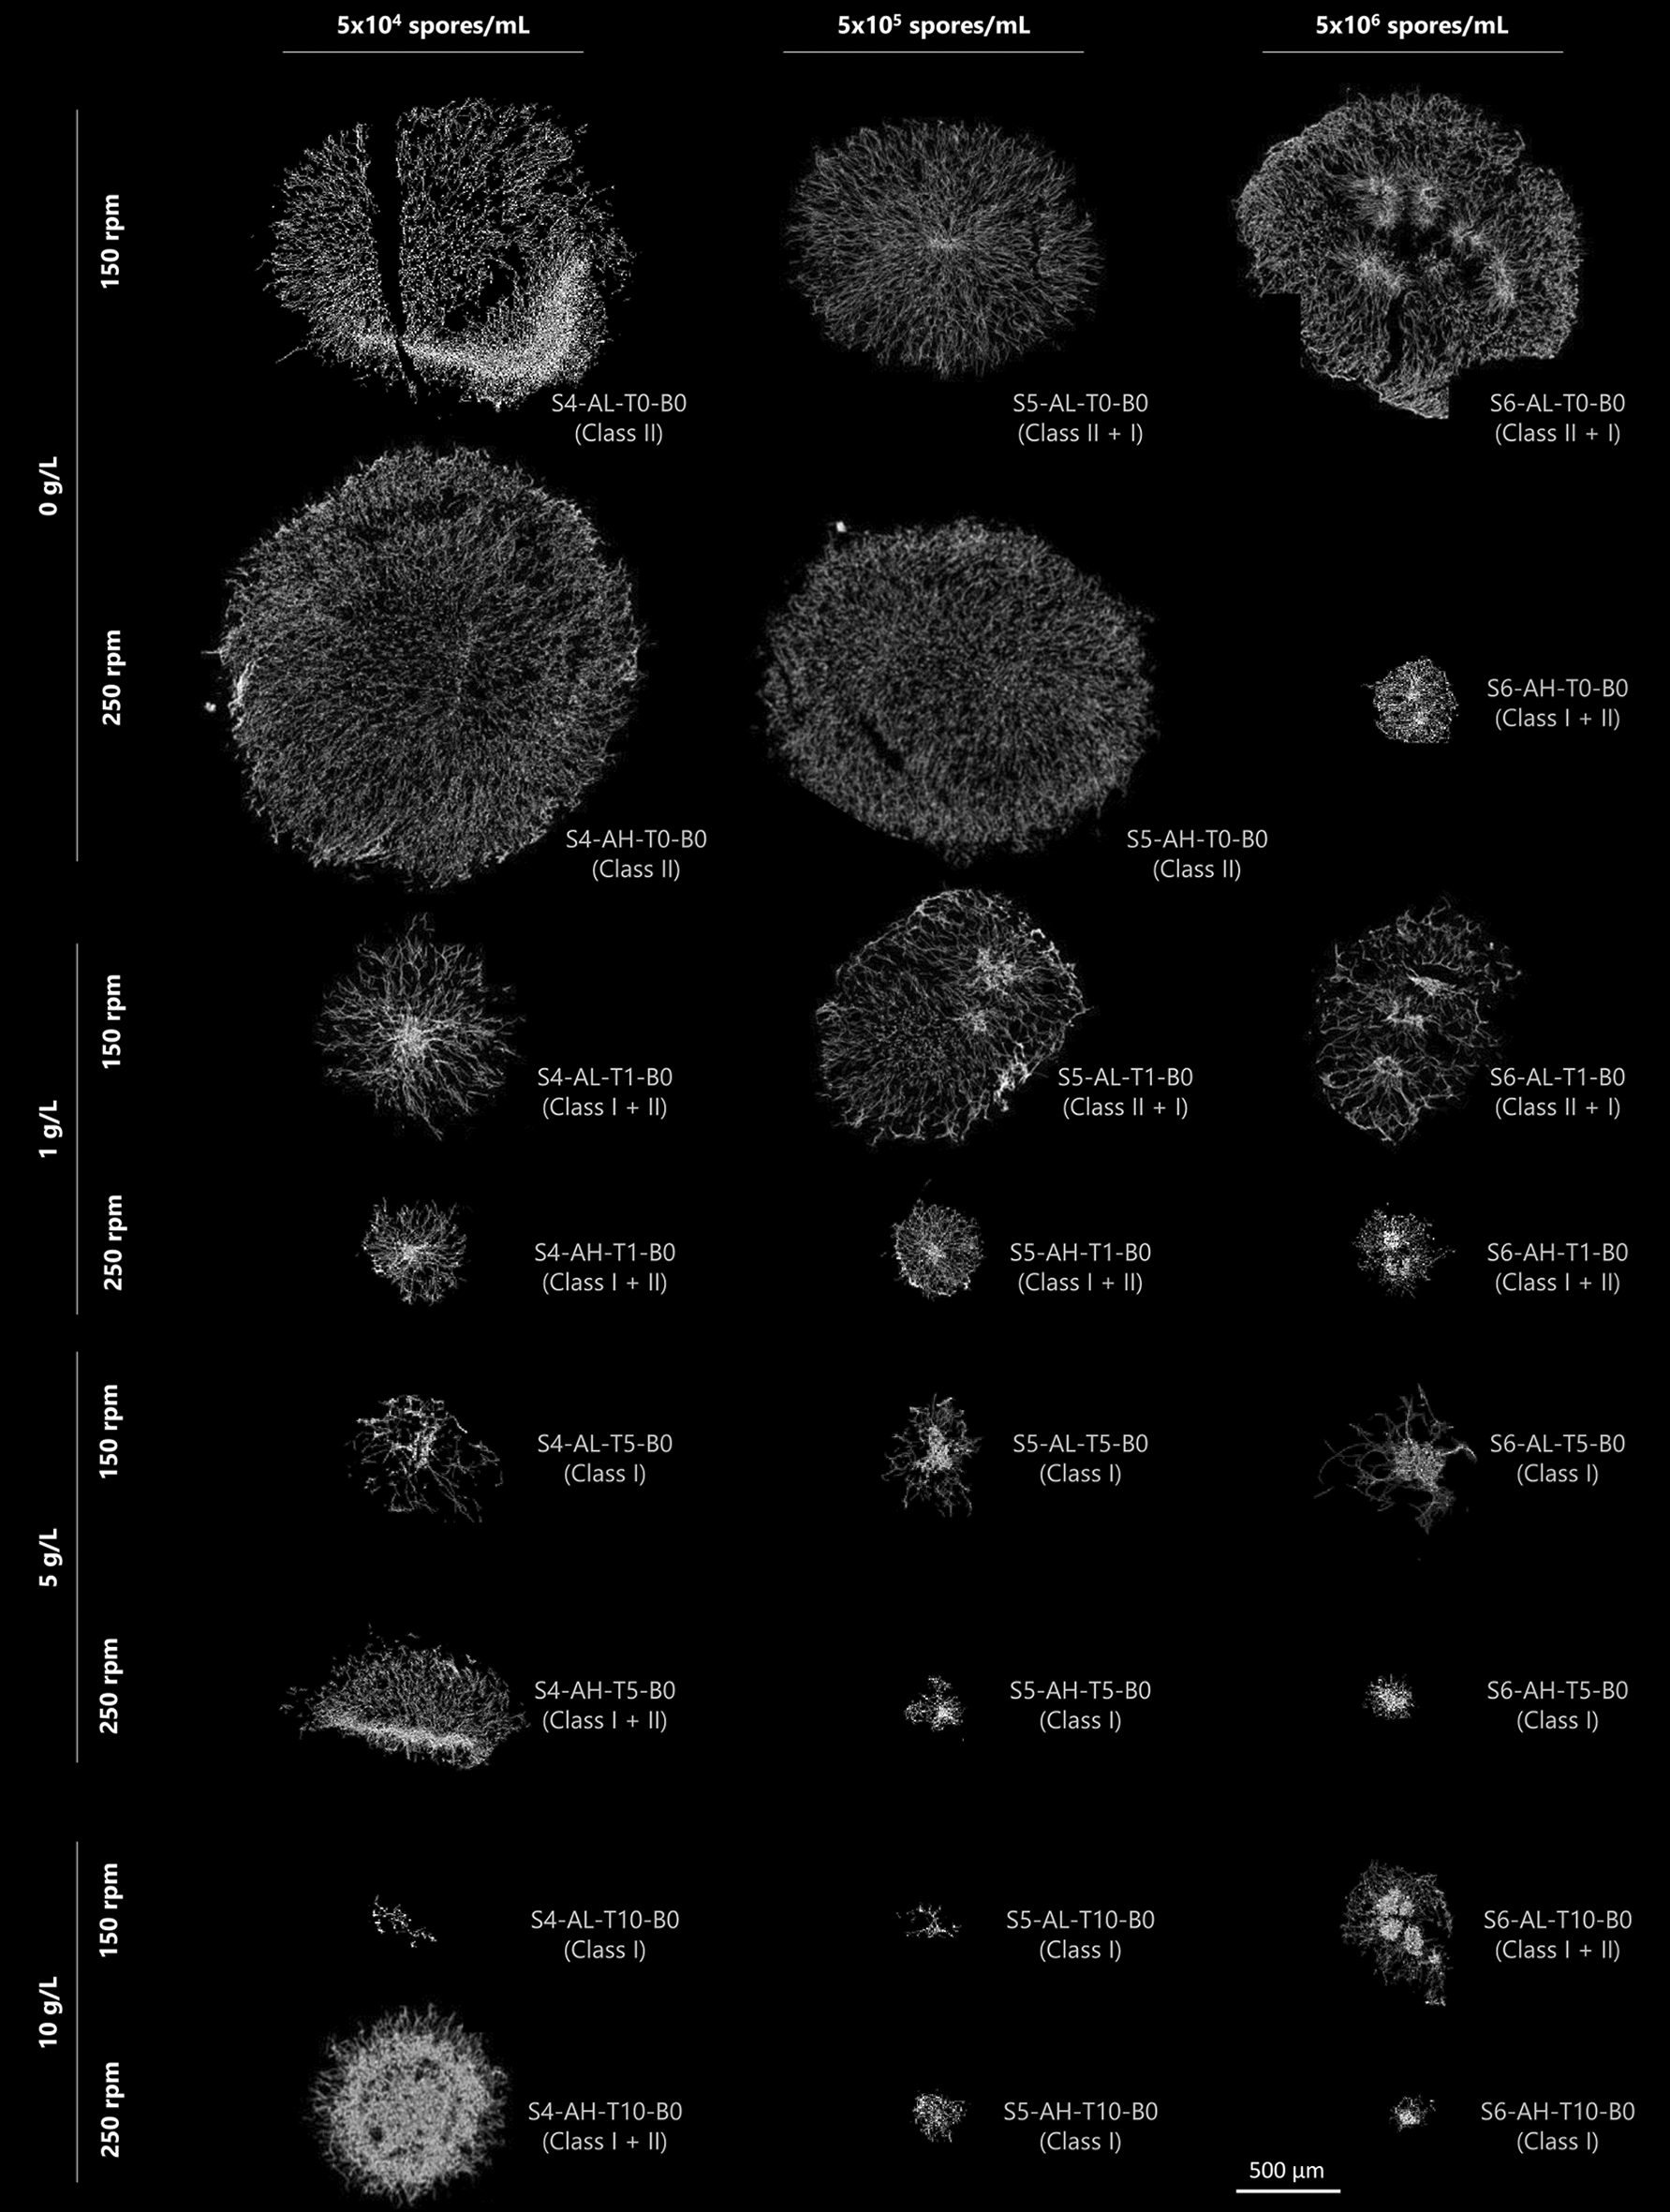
Grey level images of representative pellets from non-baffled shake flask conditions derived from synchrotron radiation-based µ-CT measurements. The images show mean intensity projections of a 25 µm-thick cross-section through the pellet’s mass centre. For each condition, the assignment of Class I, Class II and mixtures is indicated for orientation purposes, it does not represent a strict categorisation.  The g L^-1^ values indicate the talc concentration used in each cultivation condition
